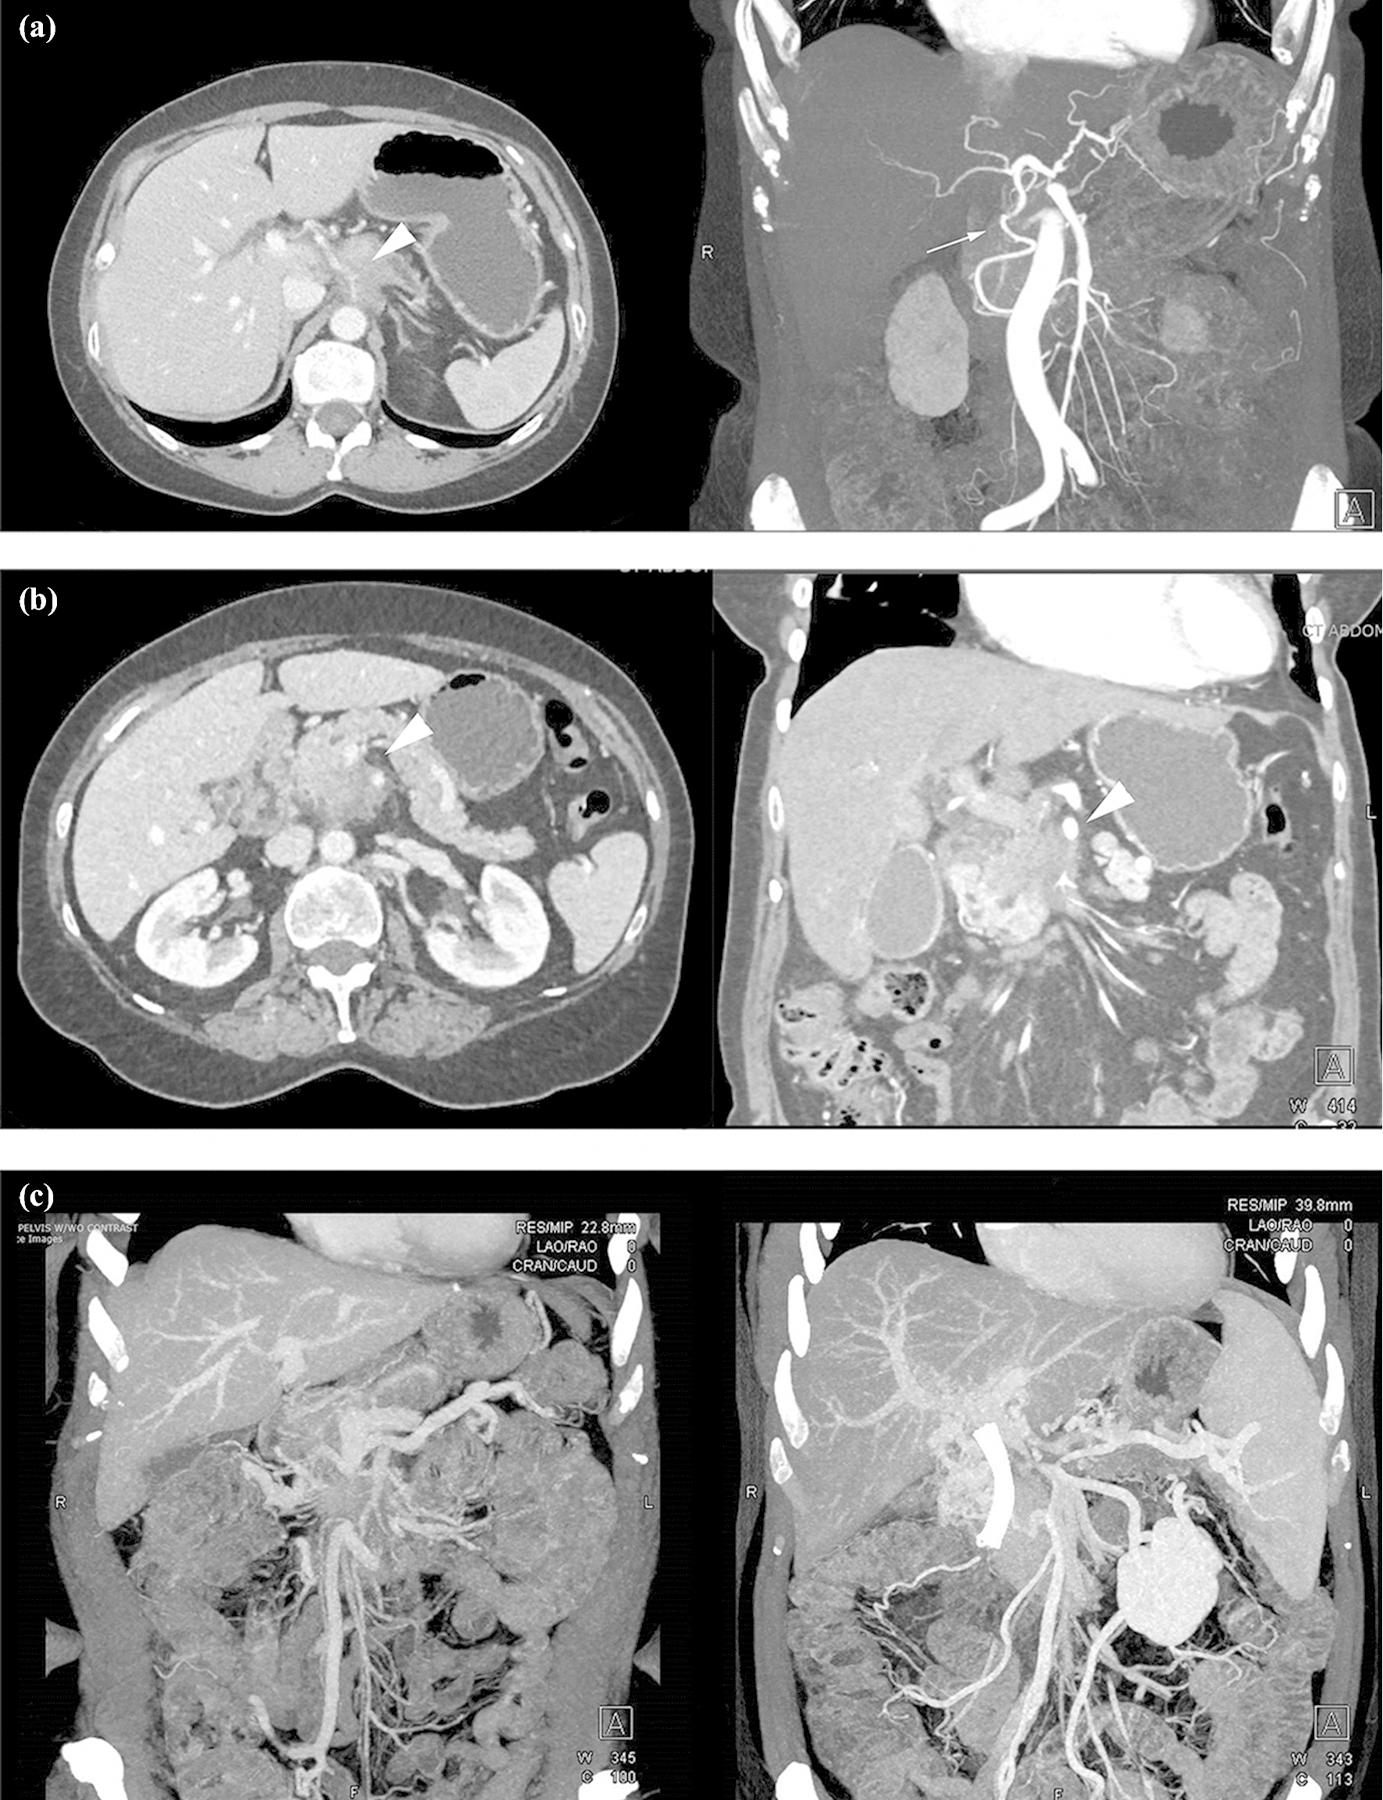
(a) CT imaging of a patient with LAPC-1: encasement of the celiac artery (arrowhead) and no involvement of the gastroduodenal artery (arrow), (b) CT imaging of a patient with LAPC-2: encasement of the SMA and SMV (arrowheads), (c) CT imaging of a patient with PDAC and non-reconstructible SMV, LAPC-3.
